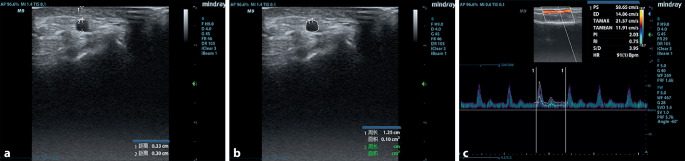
Ultrasonographic measurements: a internal diameter and depth (cm), b cross-sectional area (cm^2^, CSA), c the time average maximal velocity (TAMAX, cm/s)
